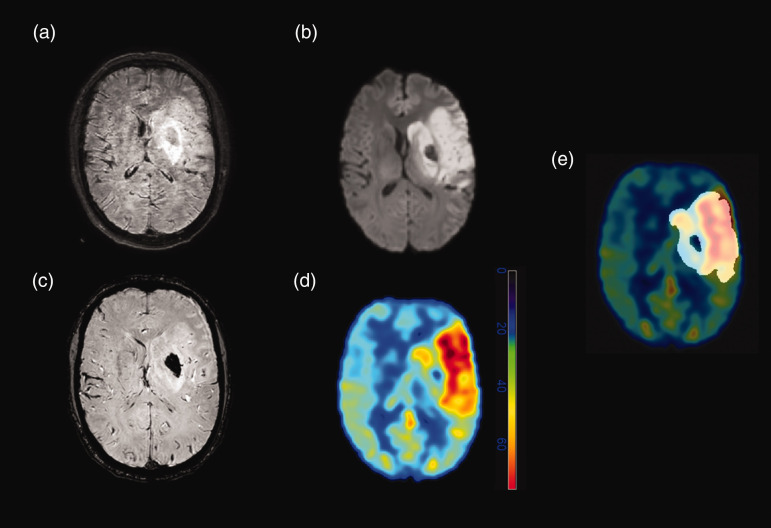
Magnetic resonance imaging (MRI) was acquired after left-sided thrombectomy of a thrombotic occlusion of the M1 segment of the middle cerebral artery in a 57-year-old male patient. (a) Fluid-attenuated inversion recovery (FLAIR) imaging (with moderate motion artifacts) shows left-sided edema in the middle cerebral artery territory. Circumscribed hypointensity is visualized within the left-sided basal ganglia, consistent with a hematoma. (b) The infarct core can be clearly seen on diffusion-weighted imaging (DWI) with similar bleeding-related, central hypointensity within the left-sided basal ganglia. (c) The hemorrhage is best seen on susceptibility-weighted imaging (SWI). (d) Around the hematoma, there is a marked increase in cerebral blood flow (CBF), as seen on the CBF map derived from pseudo-continuous arterial spin labeling (pCASL). Of note, within the hematoma, pCASL-derived CBF maps indicated decreased CBF. (e) For the analyses of the study, any bleeding was excluded from the infarct masks during image segmentations.
